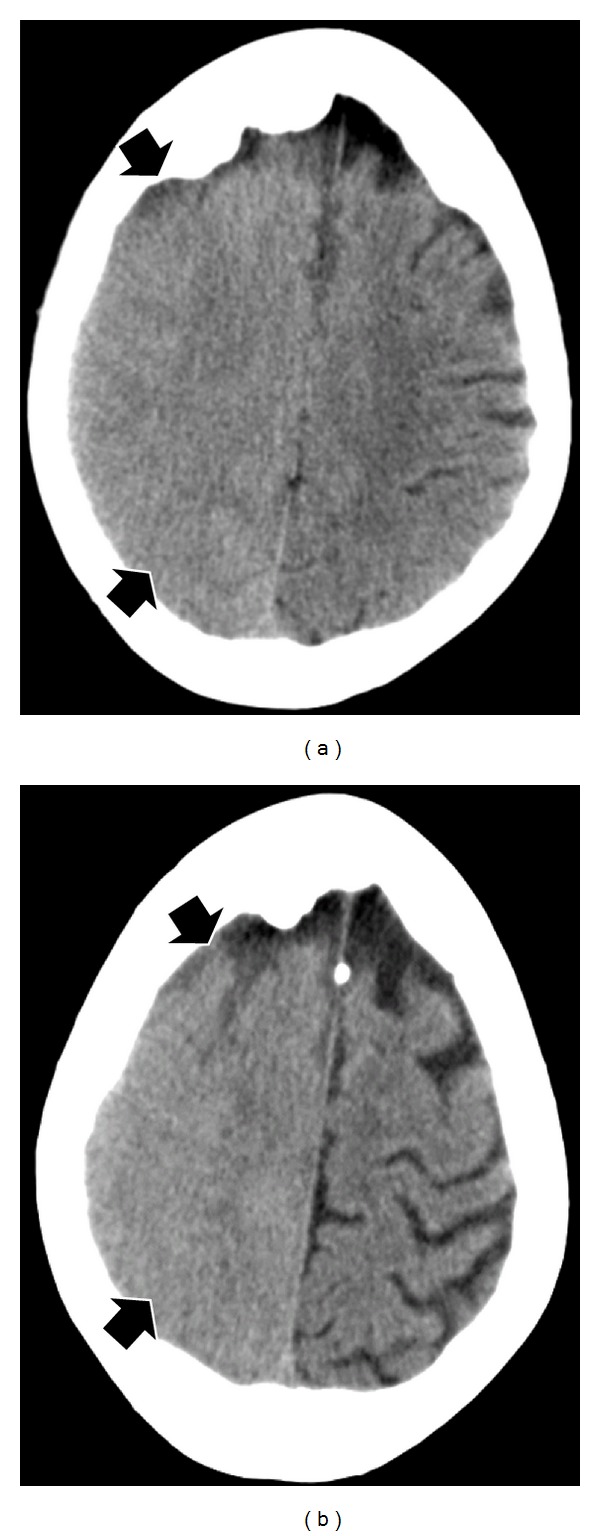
Unenhanced computed tomography obtained one hour after the onset of patient's symptoms ((a) and (b)) showed sulcal effacement in the right cerebral hemisphere due to cerebral swelling. The changes were most marked in the high frontal and parietal lobes.
